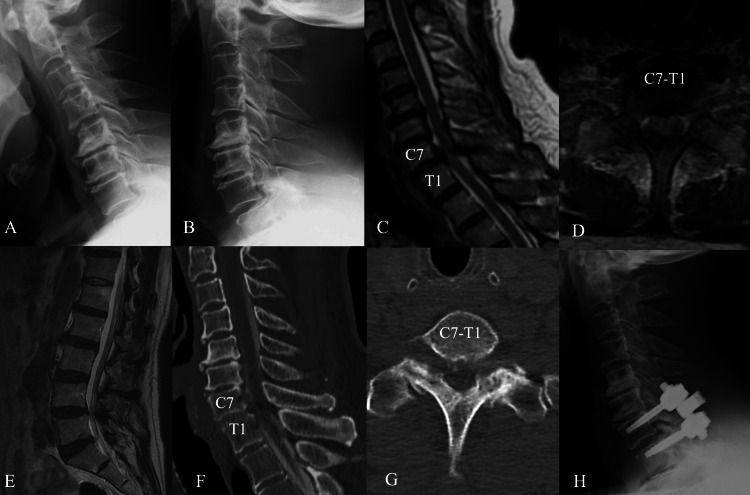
Imaging findings in Case 1 (A) Preoperative cervical spine radiograph in flexion lateral view. C7-T1 level has mild listhesis. (B) Preoperative cervical spine radiograph in extension lateral view. (C) T2-weighted sagittal magnetic resonance imaging (MRI). The spinal cord at the C7-T1 level is compressed and swollen, with mild hyperintensity. (D) T2-weighted axial MRI showing posterior compression of the spinal cord with mild listhesis of C7. (E) T2-weighted lumbar spine MRI showing mild lumbar spinal canal stenosis at L4/5. (F) Sagittal computed tomographic myelography (CTM) showing anterior-posterior compression of the spinal cord. (G) Axial CTM showing contrast thinning at the C7-T1 level and arthrosis of the C7 and T1 facet joints. (H) Lateral view of the postoperative radiograph. C7-T1 laminectomy and posterior instrumented fusion were performed.
